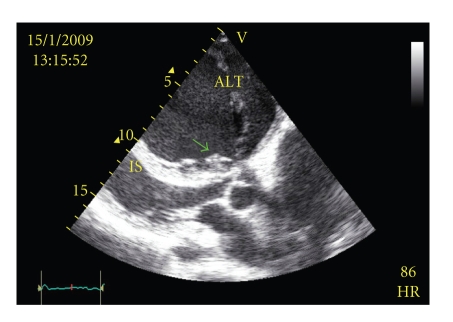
Transthoracic parasternal long-axis view demonstrating the septal leaflet apical displacement of the tricuspid valve (arrow), the “sail-like configuration” of the enlarged anterior tricuspid leaflet (ATL), and the leftward shift of the interventricular septum reducing left ventricle compliance (IS).
